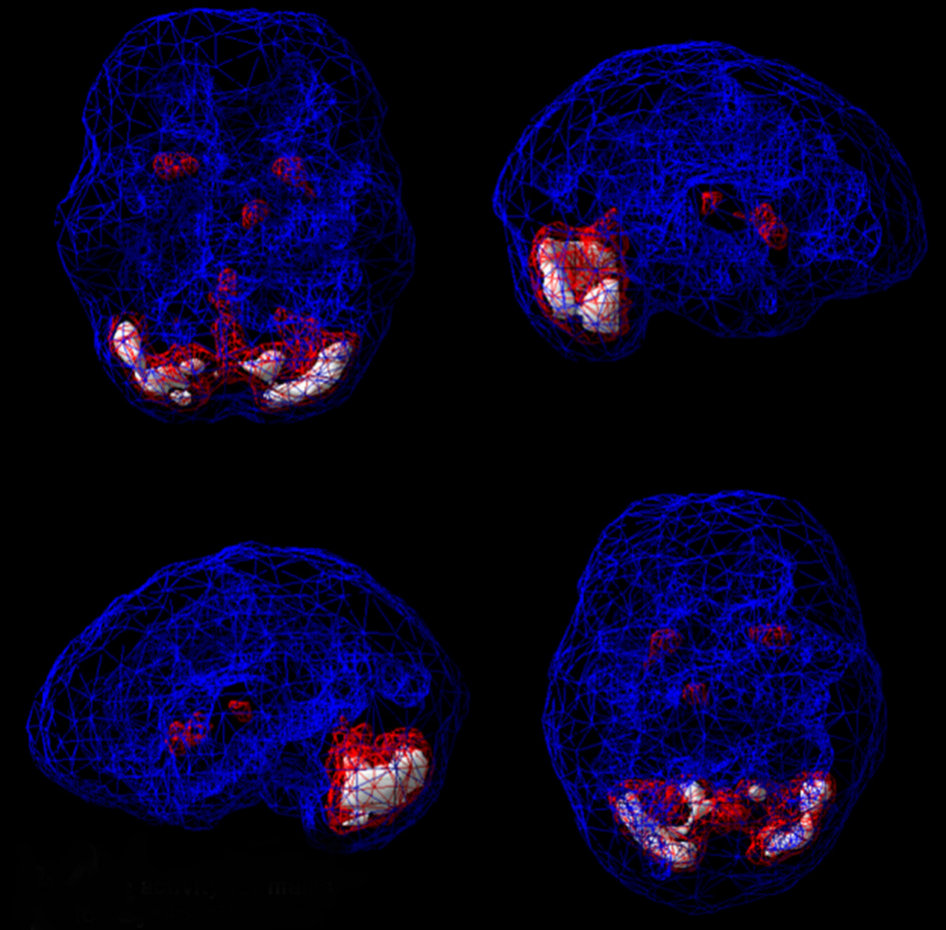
Normal active rendering. A healthy 3D active rendering of SPECT information, looking at areas of increased perfusion. Blue equals average perfusion, red equals the top 15% of perfusion, and white is the top 8%. A healthy active scan with HMPAO shows increased perfusion in the cerebellum.
